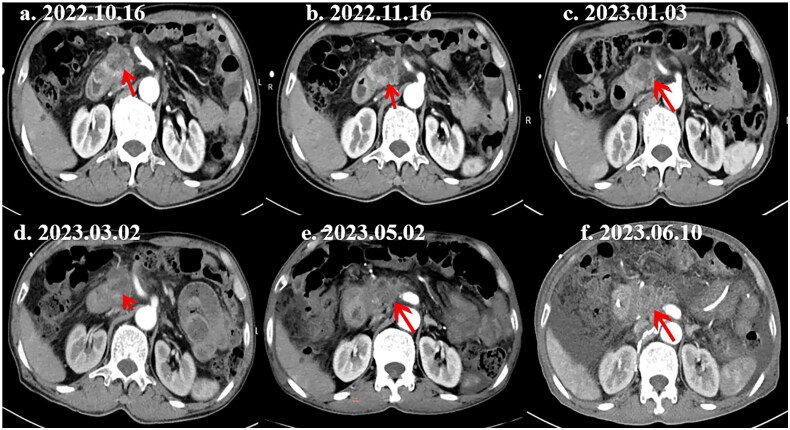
Abdominal CT imaging after treatment with lenvatinib and icaritin (red arrow: tumor sites). (a) Pre-treatment: multicystic mass in pancreatic head. (b) Post-treatment: reduced mass size, changed enhancement patterns, and enlarged lymph nodes. (c–f) During and after icaritin treatment: tumor lesions stable at 2.5–3 cm, later increasing to 3.5–4 cm.
